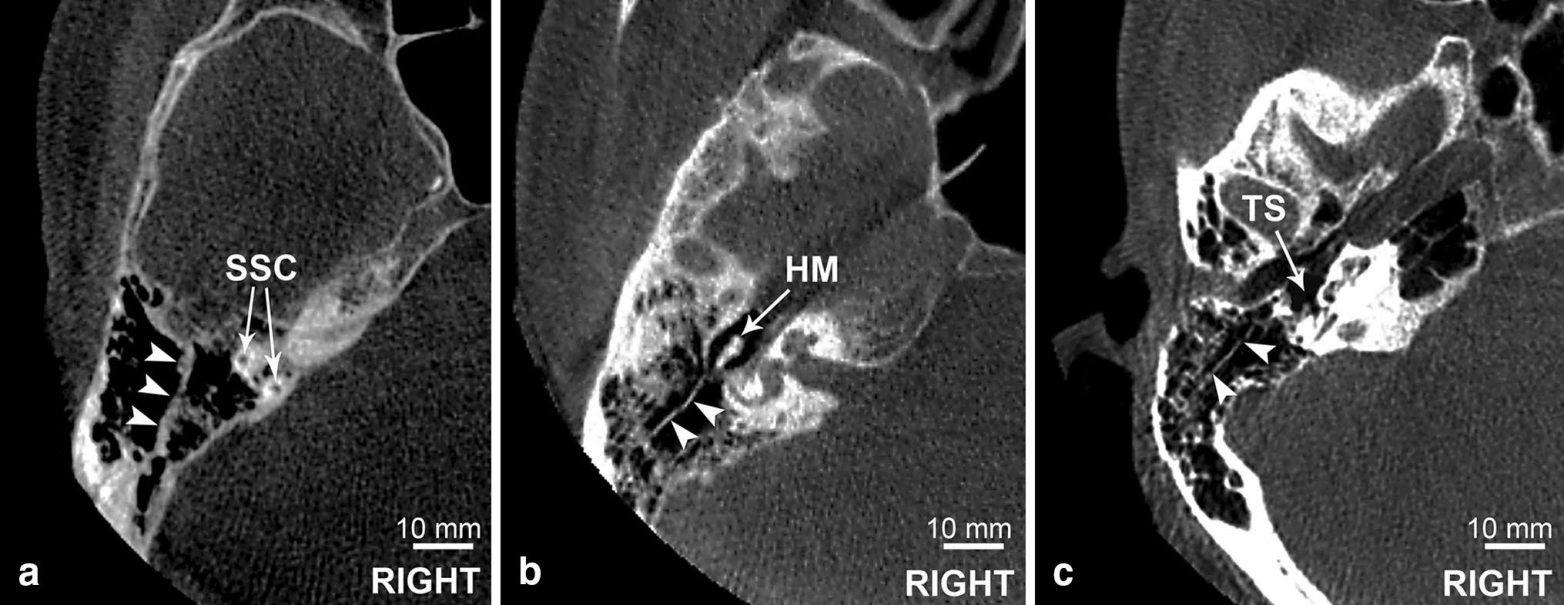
Axial CBCT scans presenting all the three landmarks used in the study. KS marked with arrowheads. a*SSC* superior semicircular canal; b*HM* head of malleus; c*TS* tympanic sinus
